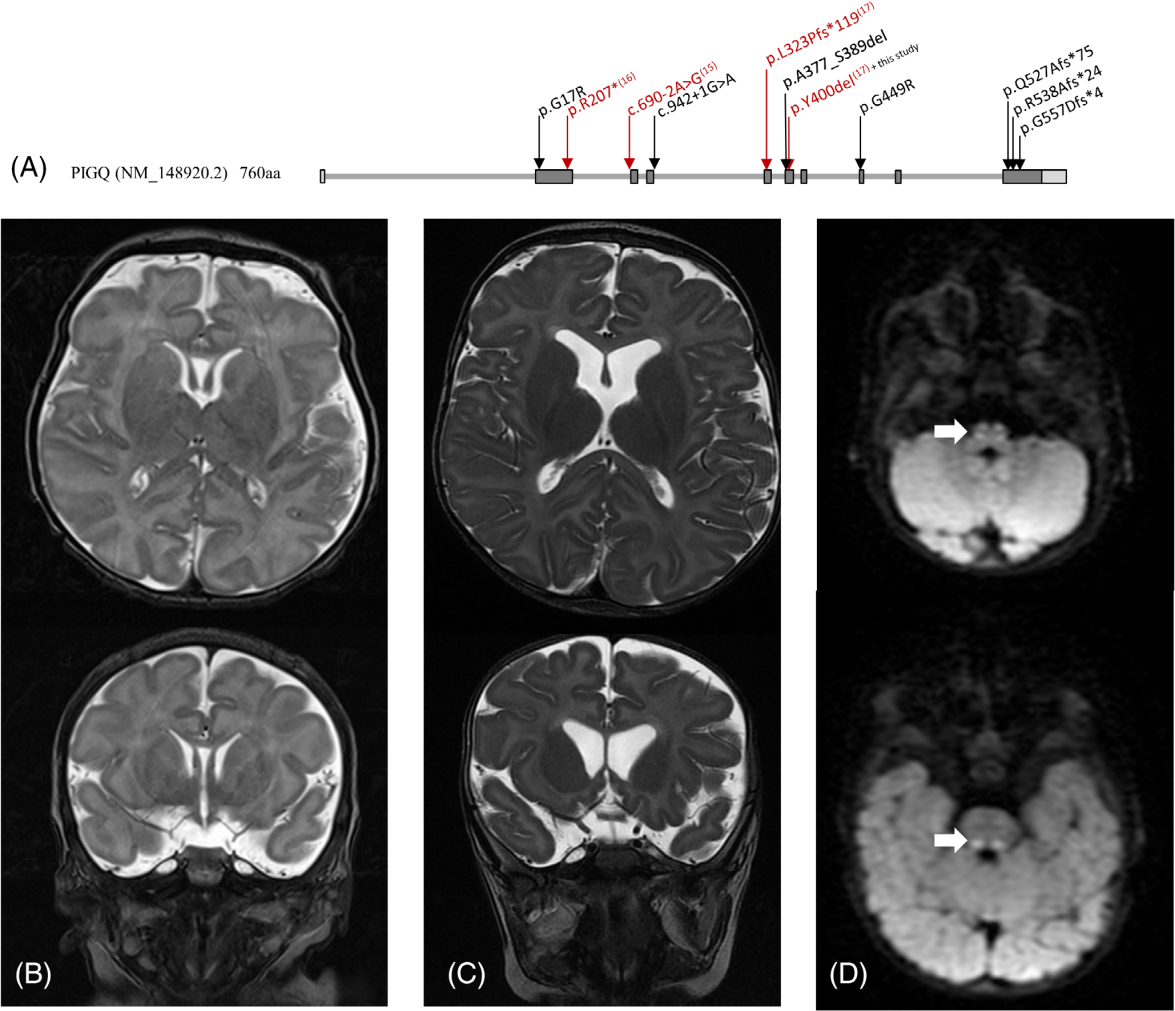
A, Variants in *PIGQ* identified in this study localized on isoform 1 (NM_148920.2) of the gene. Variants reported in previous studies are in red with corresponding reference numbers in superscript. Brain MRI of subject 4 was performed at age 9 days, B, and 7 months, C. At each age, T2‐weighted image sequences were performed with axial (top) and coronal (bottom) images shown. Brain MRI at 7 months showed progressive cortical volume loss with increased prominence of the lateral ventricles, consistent with loss of subcortical white matter volume compared to the prior study. Mild delay in myelination was apparent. D, Diffusion weighted axial images identified restricted diffusion in the medial lemniscus tracts, bilaterally
